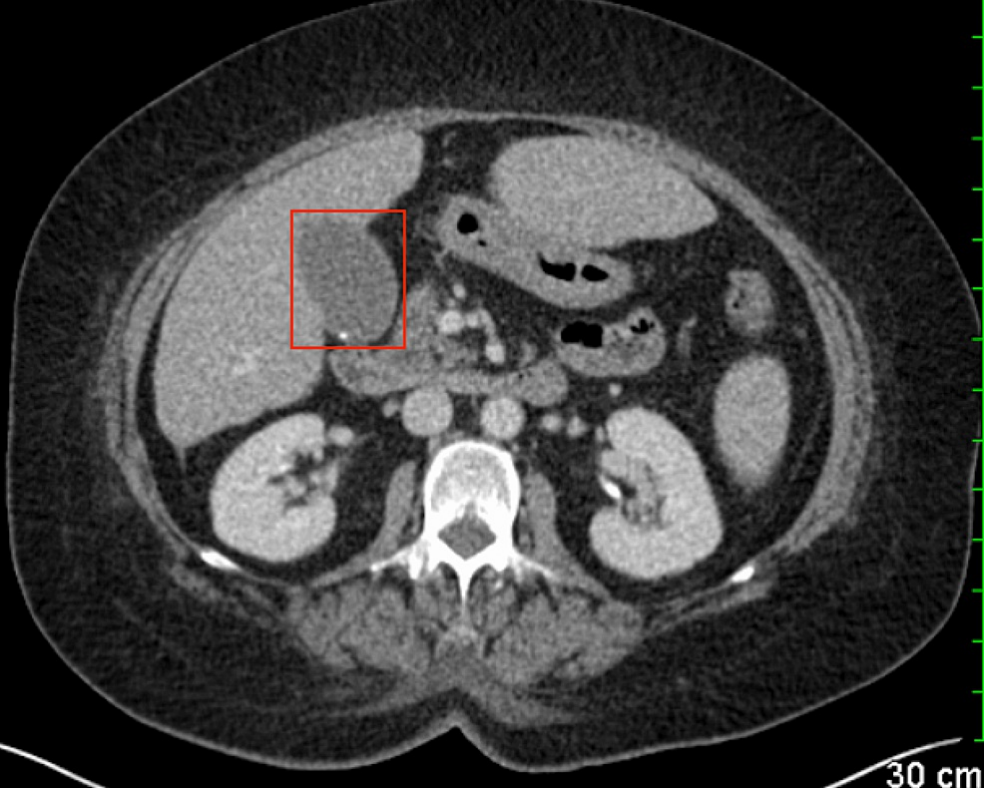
CT slice at the level of the abdomen reveals cholelithiasis. The gallbladder is outlined in the red box showing a hyperdense gallstone in the dependent portion of the gallbladder. CT: computed tomography
